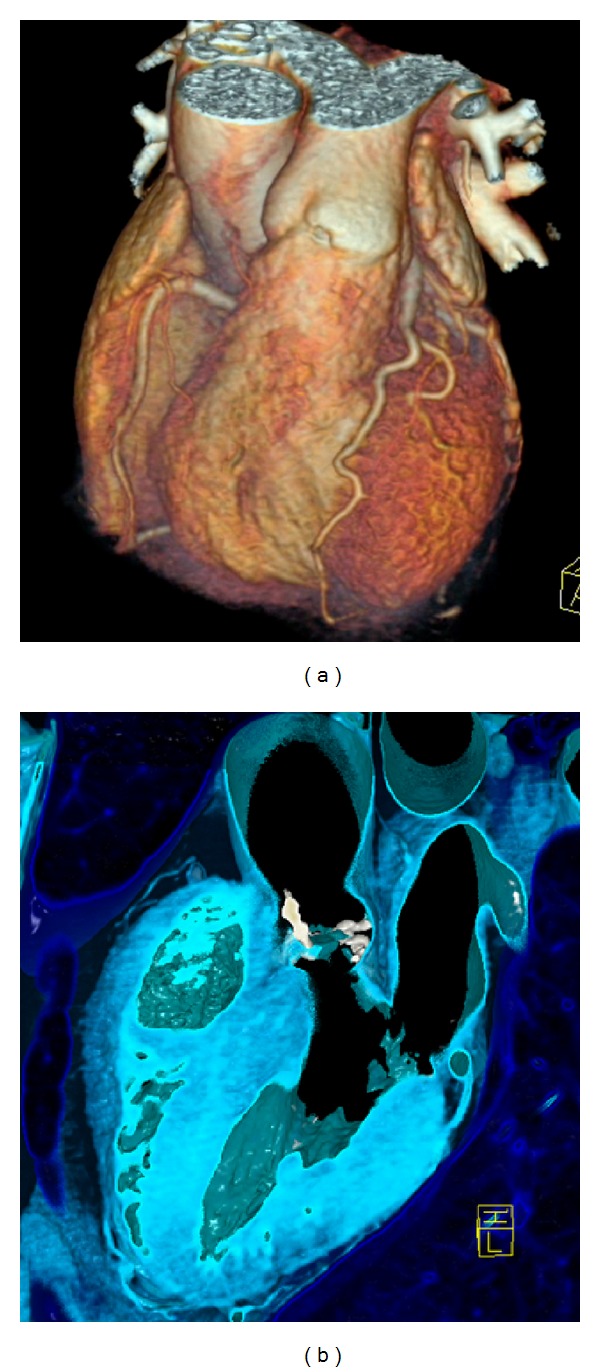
(a) *3-D VRT image of the heart by computed tomography*. The coronary arteries (LAD—left anterior descending—in the front) are shown. Cardiac valve evaluation from same CT data set is possible. (see pulmonary valve in the front). (b) *3-D image of the aortic valve. Severe calcification (white color)* of the aortic valve are pathognomonic for degenerative aortic stenosis (here shown in 3-chamber view). Further, quantification and characterization of aortic valve and annulus calcium predict complications during transcatheter aortic valve implantation (TAVI) such as postsurgery paravalvular regurgitation or annulus rupture during stent expansion.
